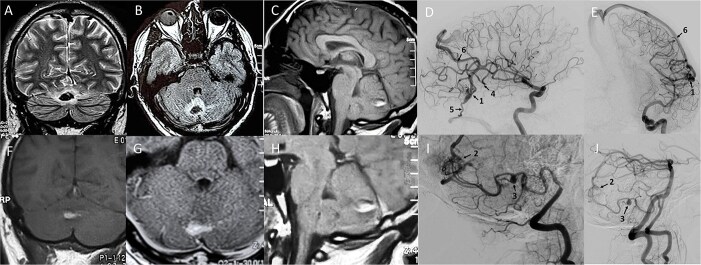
Preoperative neuroimaging. A, B, C, F, G, H - MRI of the patient’s brain in his 38, showing cerebellar vermis hematoma. D, E, I, J - digital subtraction angiography (DSA) following left internal carotid artery (D, E) and right vertebral artery injections (I, J). A - coronal T2 weighted MRI; B - axial T1 weighted MRI; C - sagittal T1 weighted MRI without contrast; D –DSA of left ICA lateral view; E - DSA of left ICA frontal view; F - coronal T1 weighted MRI with contrast; G - axial T1 weighted MRI with contrast; H - sagittal T1 weighted MRI with contrast; I - DSA of right VA lateral view; J - DSA of right VA lateral view. Black arrows (on D, E, I, J): 1 - arteriovenous malformation (AVM) of the left temporal lobe, Spetzler-Martin II; 2 - cerebellar AVM, Spetzler-Martin II; 3 - flow-related aneurysm on the afferent from the right PICA; 4 - afferent from the left middle cerebral artery; 5 - an efferent draining into the vein of Labbe; 6 - an efferent draining into the vein of Trolard.
